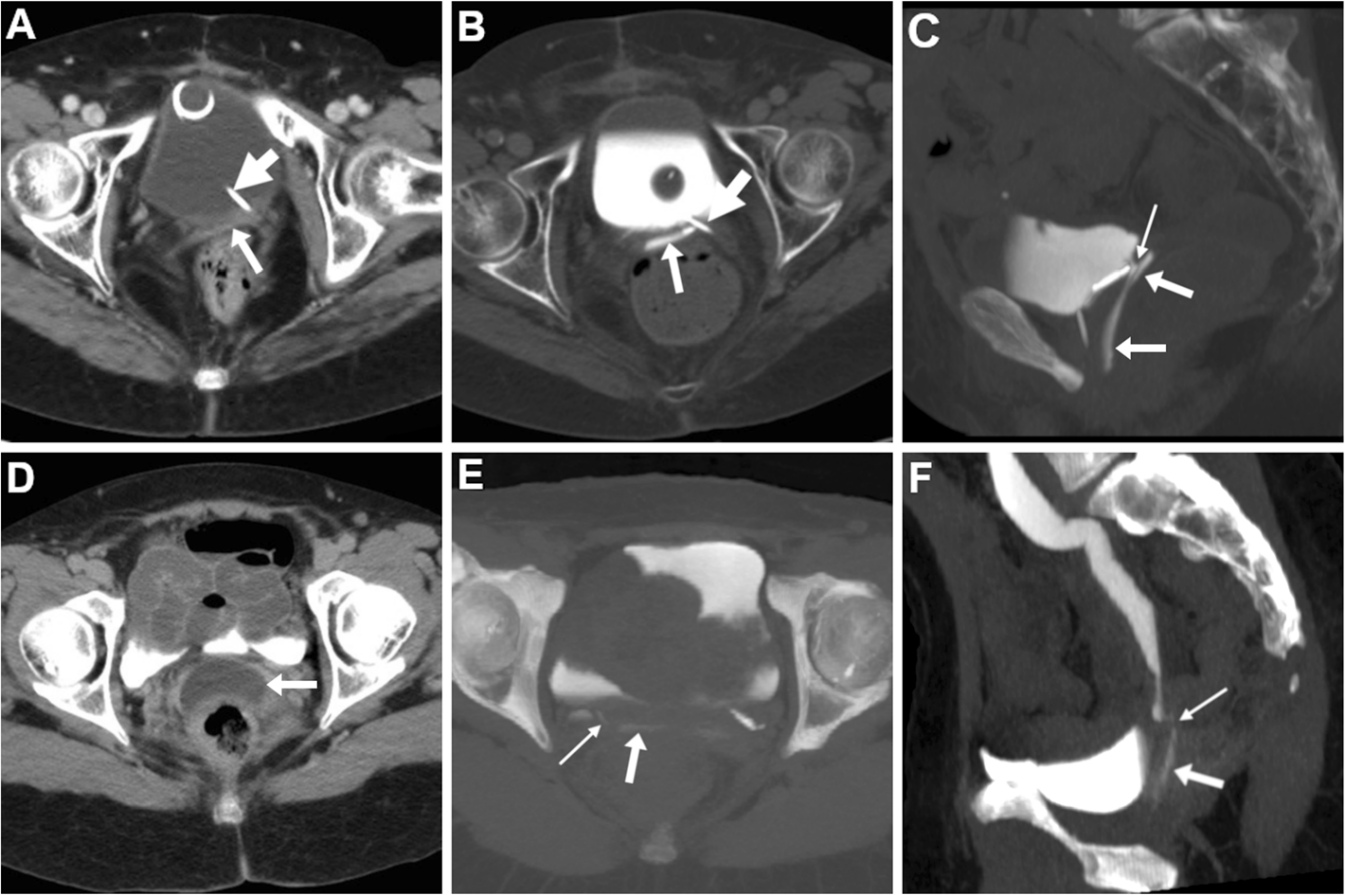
Two cases of iatrogenic urinary VF. a–c After recent surgery for recurrent endometrial carcinoma, CT-urography (note ureteral stent indicated by thick arrows) shows left lateral retraction and opacification of the vagina (arrows) through a short vesico-VF (thin arrow in c) [Adapted from Open Access Ref. no. [25]]. d–f Following radical hysteroannessectomy for endometrial carcinoma, CT-urography shows marked fluid-filled dilatation of the vagina (arrow in d). On ultra-delayed (30 min) acquisition (e, f), some opacified urine flows into the vagina (arrows) through a leaking uretero-VF (thin arrows)
